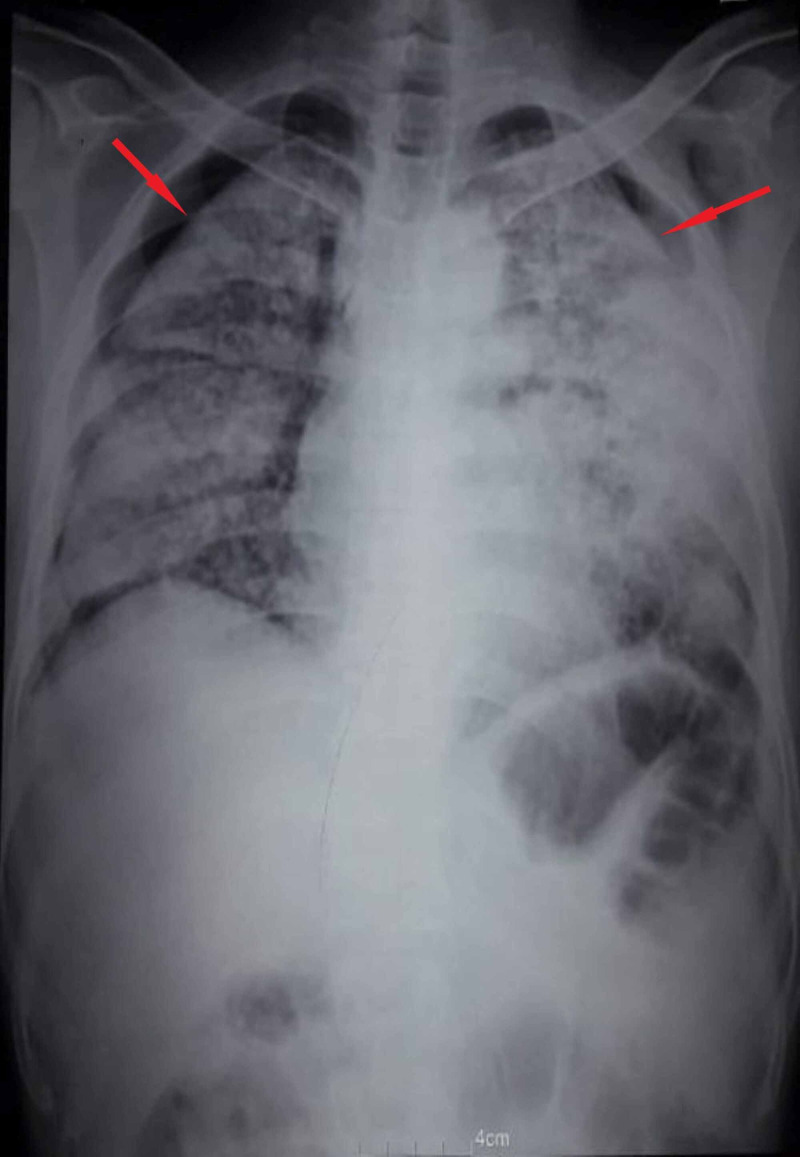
Chest X-ray of bilateral pneumothorax
